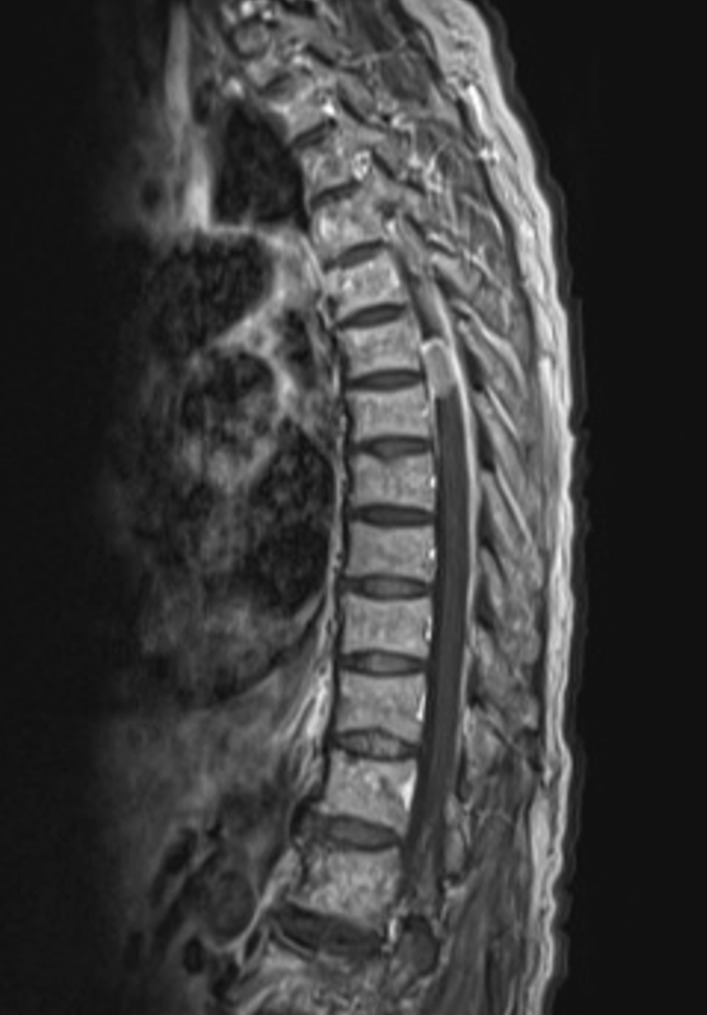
A 61-year-old woman presented with a 4-month history of numbness and pain in both lower limbs that were nonresponsive to conservative treatments. Preoperative sagittal magnetic resonance image demonstrated a large oval-shaped mass at T6-7 level
